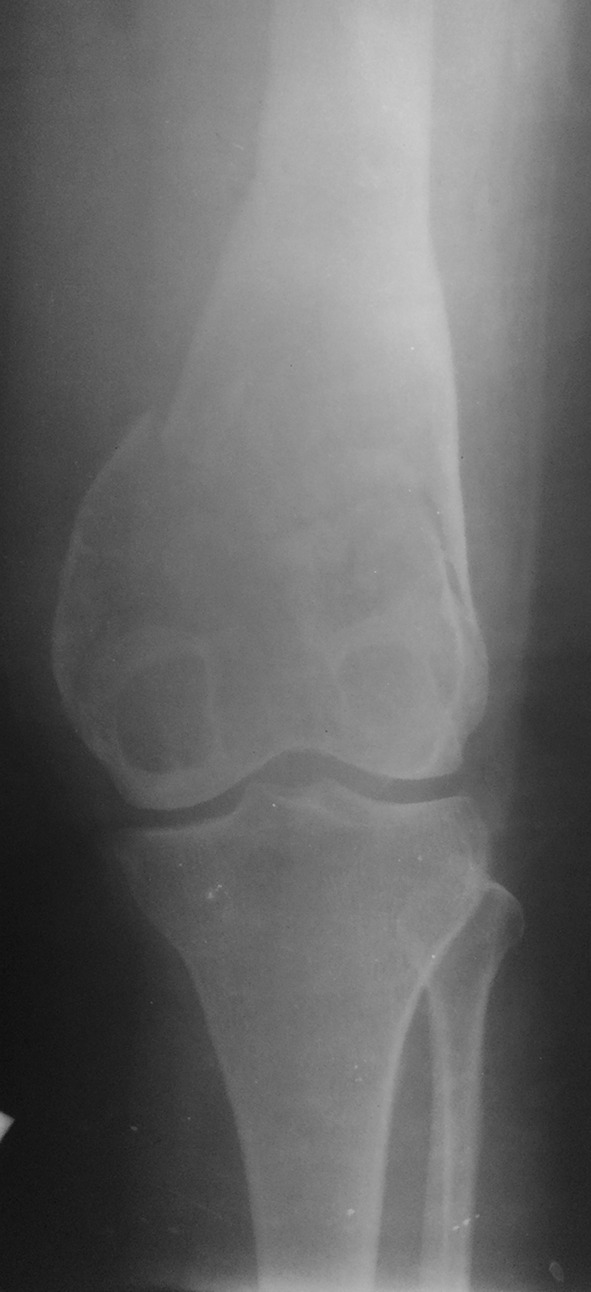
Radiograph showing an osteolytic lesion in the distal femur with an associated pathological fracture
